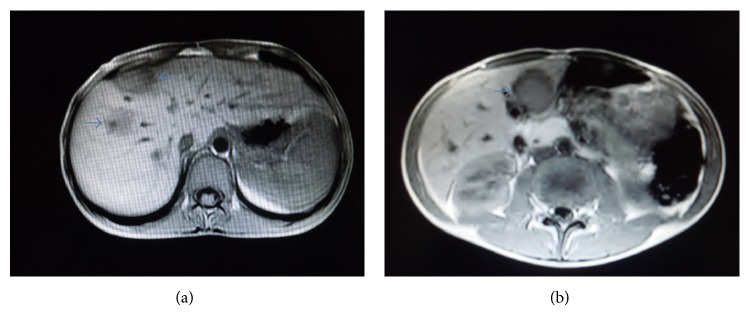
T1 (a) and T2 (b) weighted magnetic resonance imaging shows multiple lesions in the liver.
